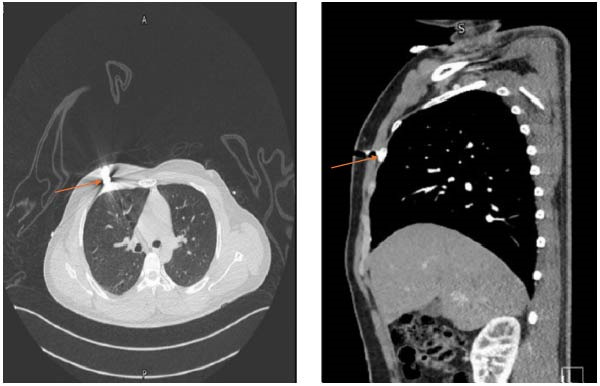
Chest CT scan showing retained bullet.
